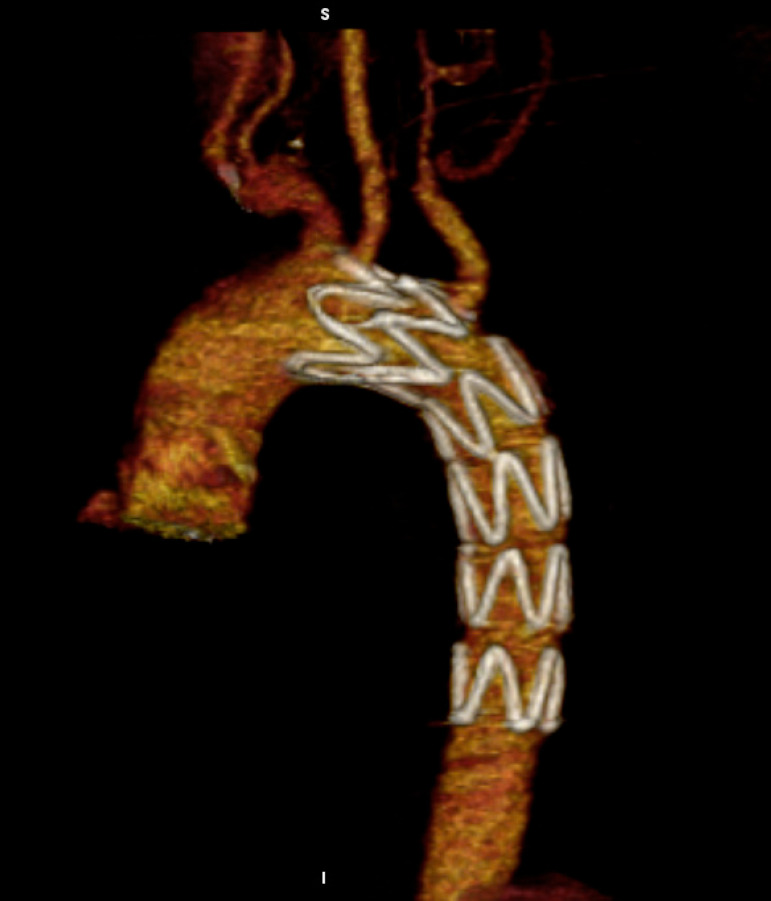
Volume-rendered three-dimensional follow-up computed tomography angiography imaging showing the endograft deployment in zone 2 and the exclusion of primary thoracic aortic mural thrombus.
